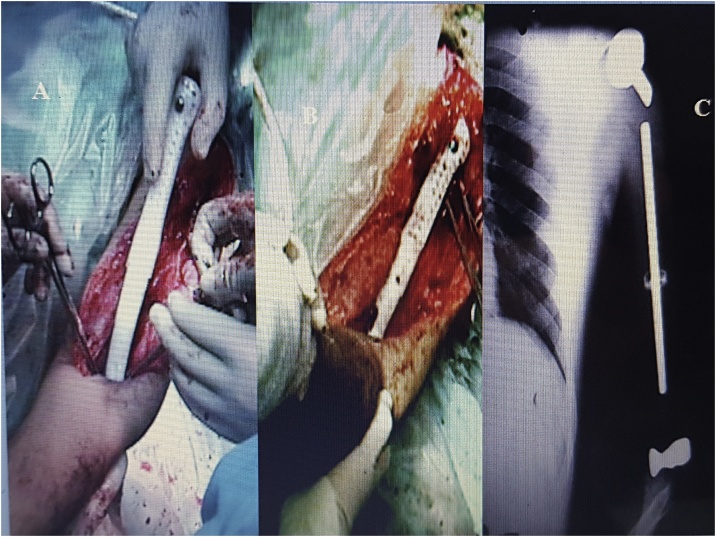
A, B total humerus prosthesis. C postoperative X-rays.
